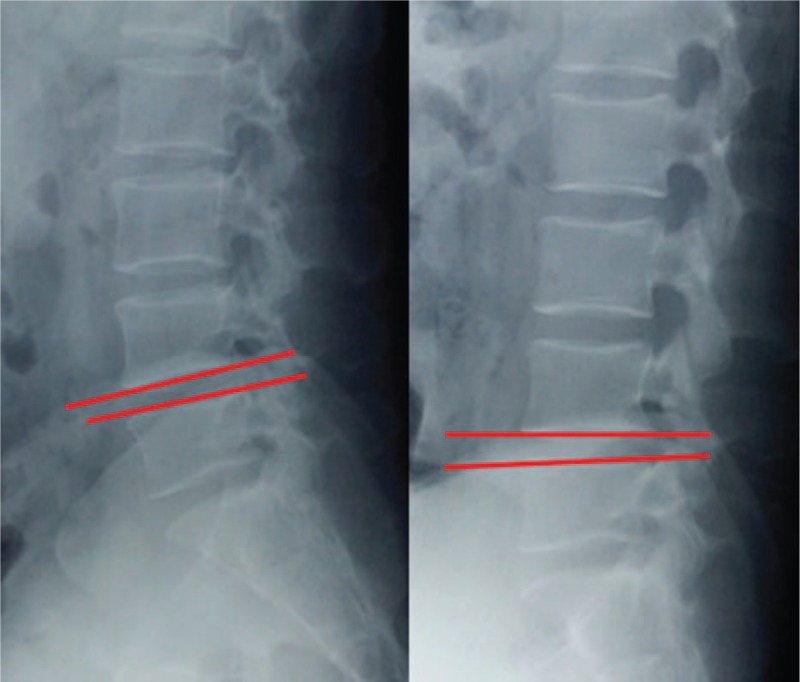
Angular motion was measured between the inferior end plate line of the upper vertebral body and superior end plate line of the lower vertebral body on flexion and extension lateral radiographs.
